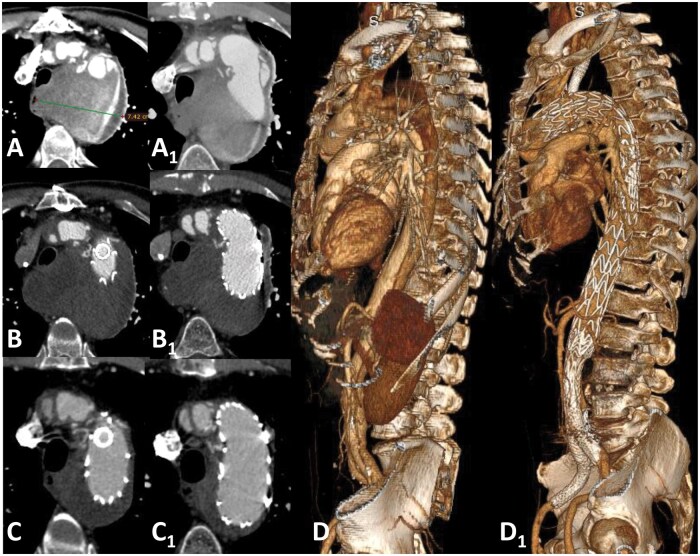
Preoperative CT-A (A–C) and 12 months follow-up CT-A (A_1_, B_1_, C_1_) of the descending thoracic post-dissection aneurysm (case #26) showing the complete shrinkage of the sac. Preoperative (D) and 12 months follow-up (D_1_) volume-rendering 3D CT-A. CT-A: computed tomography-angiography.
